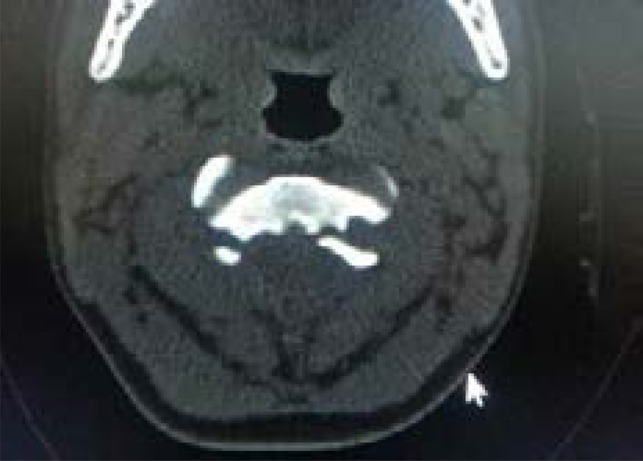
Axial CT scan of patient showing fracture of C2 traversing through the transverse foramina of C2.
